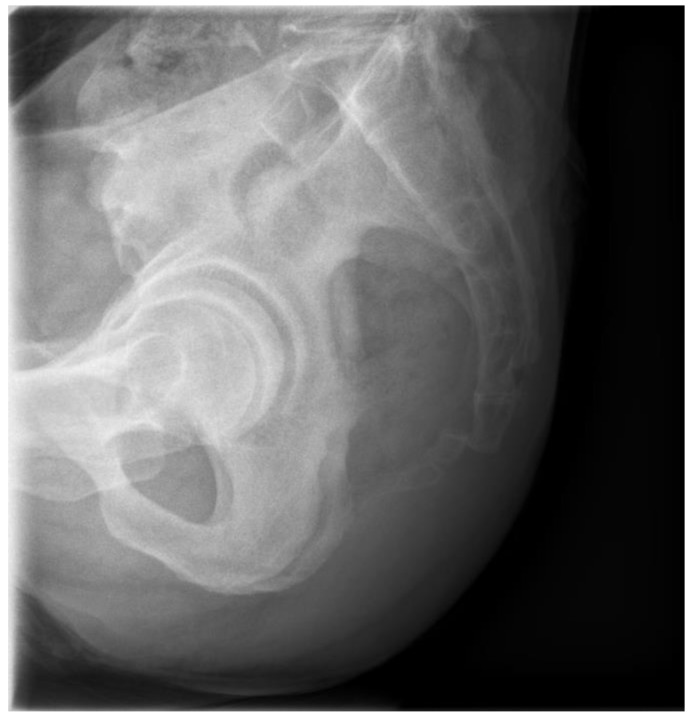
Initial plain lateral radiography shows anterior dislocation of the coccyx.
